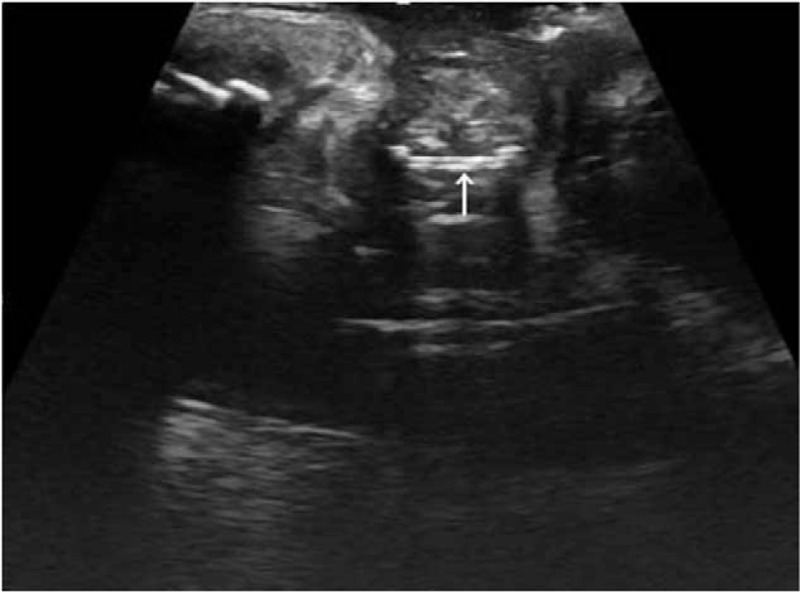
A transabdominal B ultrasound image showed a 59 × 55 × 23 mm fusiform region with increased signal intensity in the vagina followed by a sound shadow.
